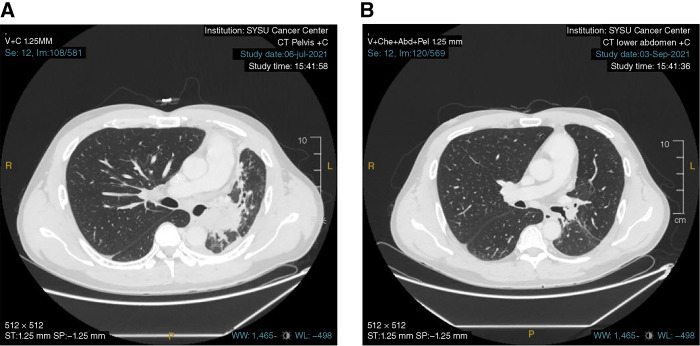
Typical radiographic images of the patient who achieved PR. A and B, A 41-year-old patient with HNSCC achieved PR after two cycles of izalontamab 16 mg/kg one dose per week treatment. SYSU Cancer Center, Sun Yat-sen University Cancer Center.
